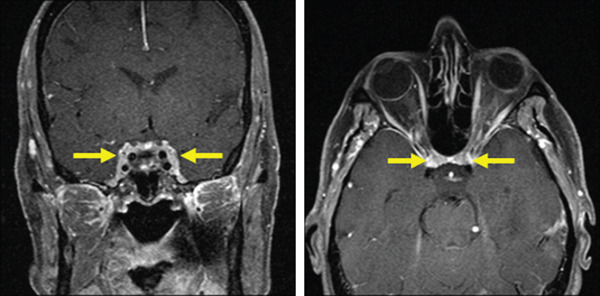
On the left panel is a coronal post‐gadolinium T1 magnetic resonance imaging (MRI) showing abnormal enhancement of both cavernous sinuses (bold arrows) as seen in meningiomatosis. On the right is the axial T1 post‐gadolinium showing bilateral peri‐optic canal meningiomas (bold arrows) straddling both optic nerves not previously reported on prior imaging.
